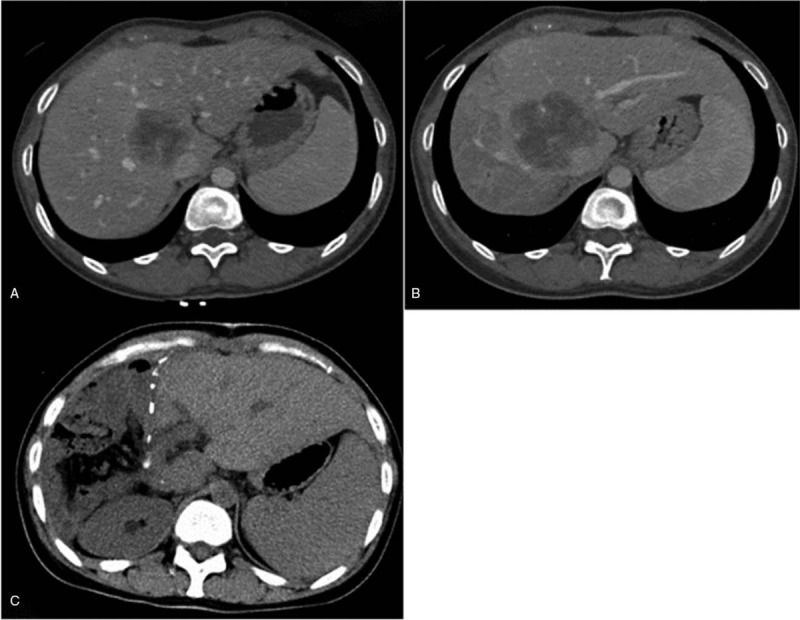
Contrast-enhanced CT image of the abdomen in a 61-year-old female with a hepatocellular carcinoma. (A) Focus on the right hepatic lobe (FLR/TELV, 36%). (B) Contrast-enhanced CT image obtained after TPVE, demonstrating hypertrophy of the FLR (FLR/TELV, 40%). (C) CT image obtained after the right hepatectomy.
